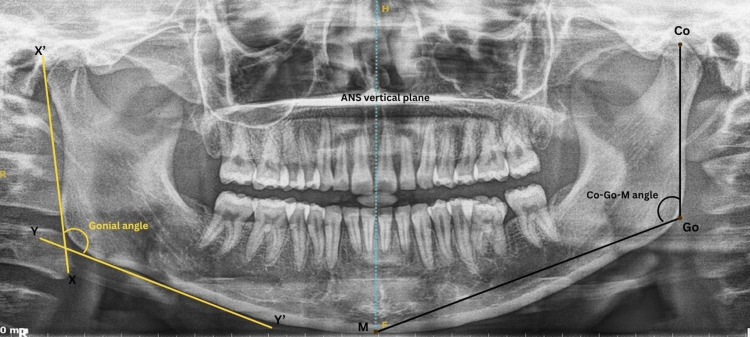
Angular parameters traced on an orthopantomogram The gonial angle is formed between the ramal plane (XX') and the mandibular plane (YY'). The Co-Go-M angle is formed by the points Co, Go, and M. Co: Condylion; Go: Gonion; M: midpoint on the inferior border of the mandible at its intersection with the anterior nasal spine vertical plane This figure represents the original orthopantomogram of the patient, obtained with the patient's permission, from the study.
